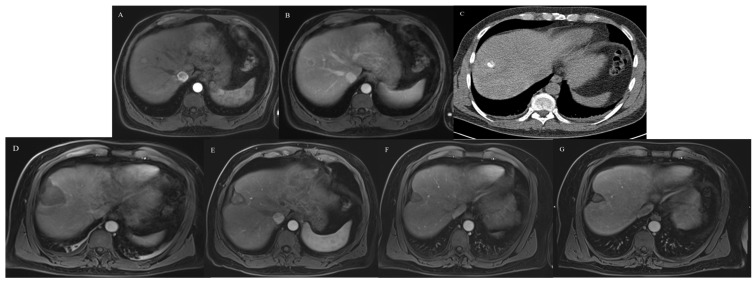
49-year-old male patient with HCC lesion in liver segment VIII treated by one session of lipiodol-based TAE, followed by CT-guided MWA. (A,B) Contrast-enhanced MRI scan shows typical pattern of HCC. (C) Unenhanced CT scan 24 h after TAE shows a compact accumulation of lipiodol within the tumor. (D) Contrast-enhanced MRI scan 24 h after MWA. (E) Contrast-enhanced MRI scan at 9-month follow-up. (F) Contrast-enhanced MRI scan at 2-year follow-up. (G) Contrast-enhanced MRI scan at 4-year follow-up shows only a small residue of ablation area without occurring of LTP.
